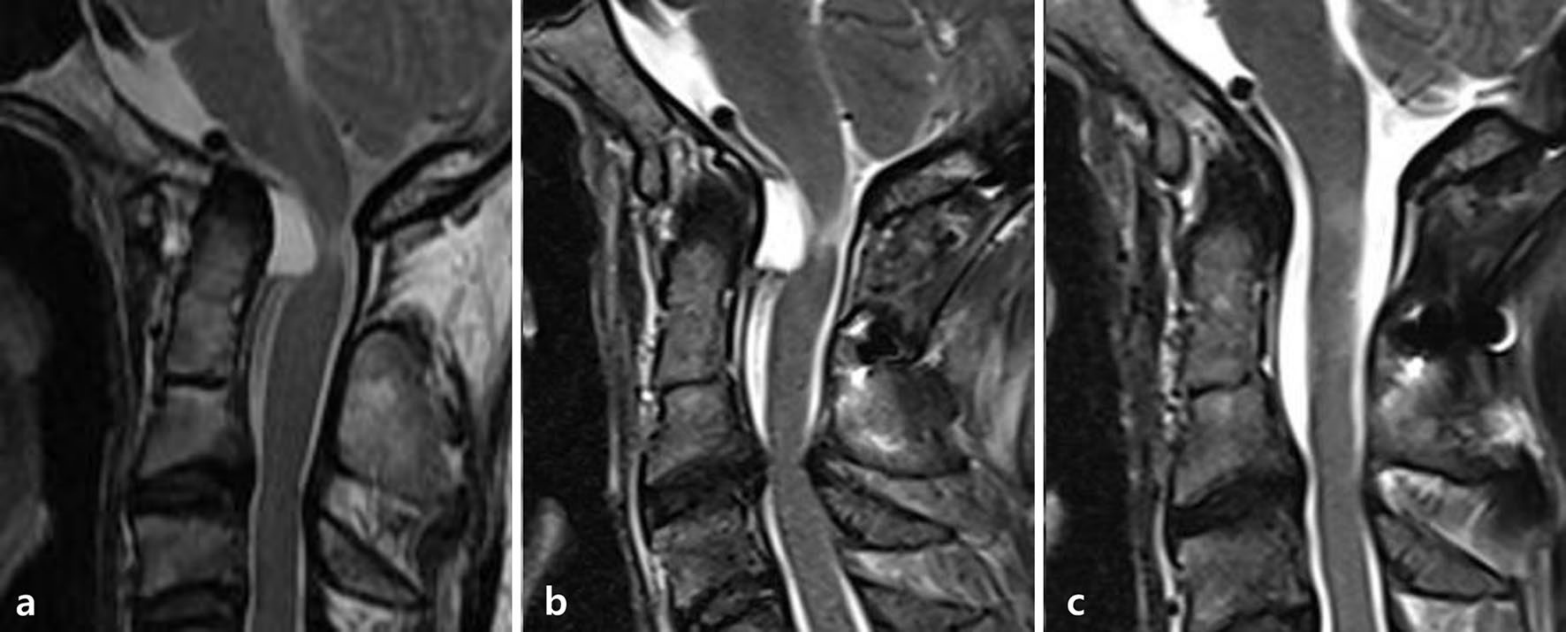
(a) Preoperative dynamic X-ray shows no instability at C1-2. Preoperative MRI shows R-OM with spinal cord compression. (b) C1-2 instability was observed at 18 months after decompression surgery. The R-OM size increased at the 18-month follow-up MRI examination. (c) The R-OM size decreased at 6 months after fusion surgery. *MRI* magnetic resonance imaging, *R-OM* retro-odontoid mass.
